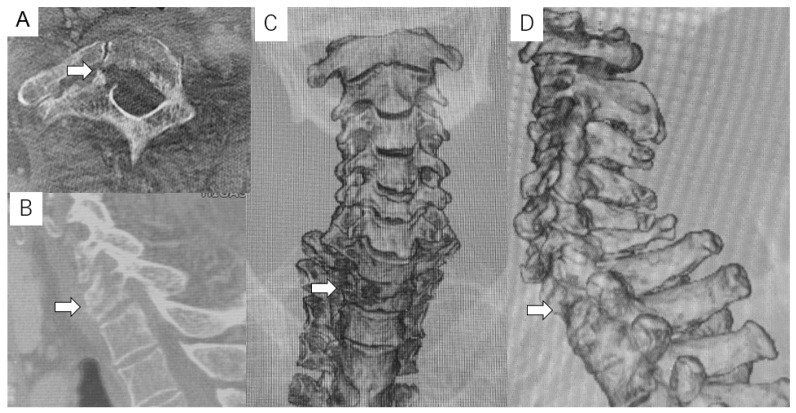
Computed tomography (CT) (A) Axial view—an osteolytic lesion was identified in the right T1 pedicle; (B) sagittal reconstruction view—the T1 vertebra collapsed; (C) anterior 3D—the right side of the T1 vertebra collapsed; and (D) lateral 3D. White arrows indicate tumor location.
